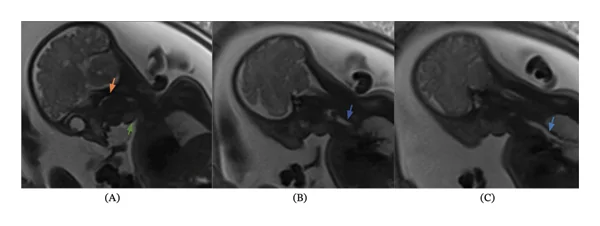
Sagittal T2‐weighted MRI images. (A) Severe micrognathia/agnathia with retrognathia (green arrow), severe narrowing/obliteration of the oropharynx and hypopharynx, and glossoptosis with posterior displacement of the tongue toward the pharyngeal wall (red arrow). The distal airways remain fluid filled (blue arrow). (B, C) Normal fluid‐filled distal trachea and bronchi, effectively excluding congenital high airway obstruction syndrome (CHAOS).
